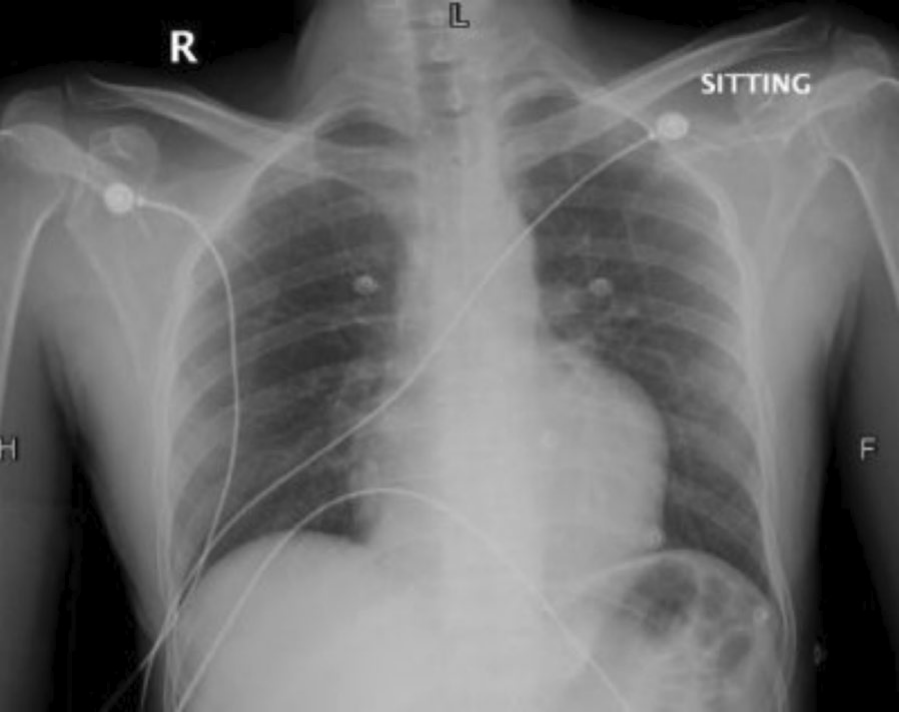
Chest X-ray shows slightly irregular and rounded left heart border
